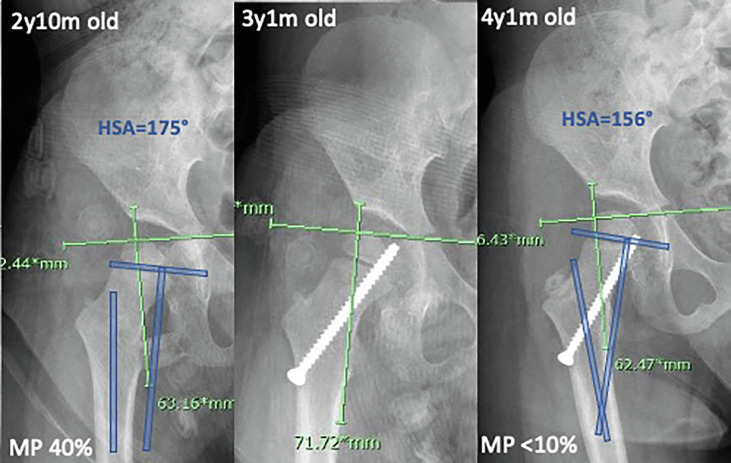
Radiographs showing progressive hip displacement in a child with hypotonic CP (without adductor contractures) treated with guided growth of the proximal femur. Note the reduction in head-shaft angle (HSA, blue lines) and reduction in migration percentage (MP) post-operatively. Change in MP occurred rapidly, likely due to the relatively low pre-operative MP and high growth velocity of the proximal femur at this young age.
